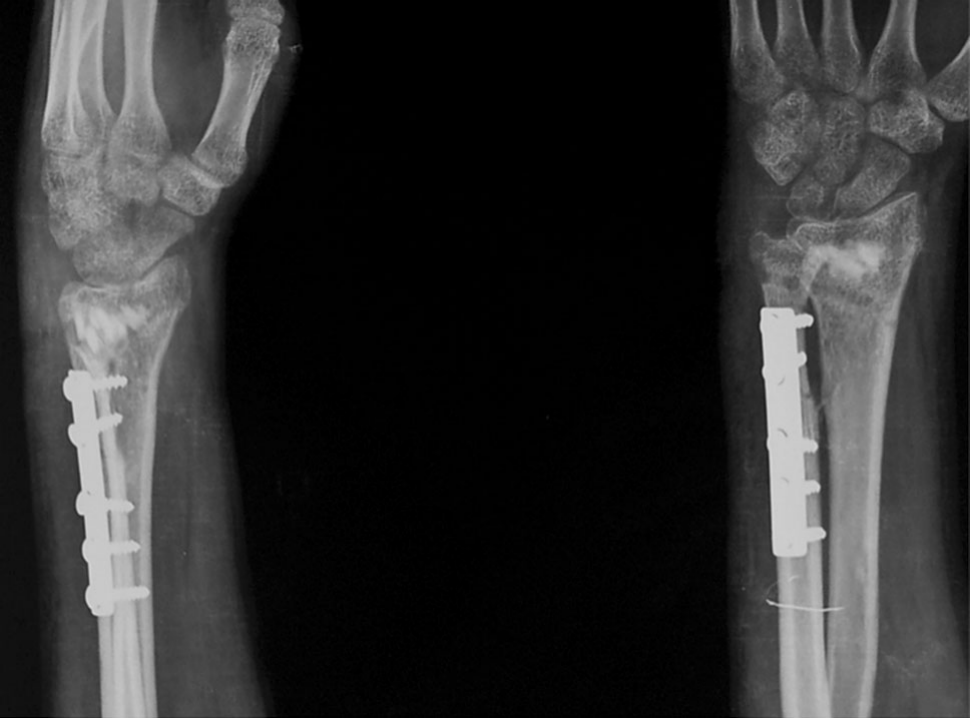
Radiographs 6 weeks after the surgery, after removal of K wires and screw
